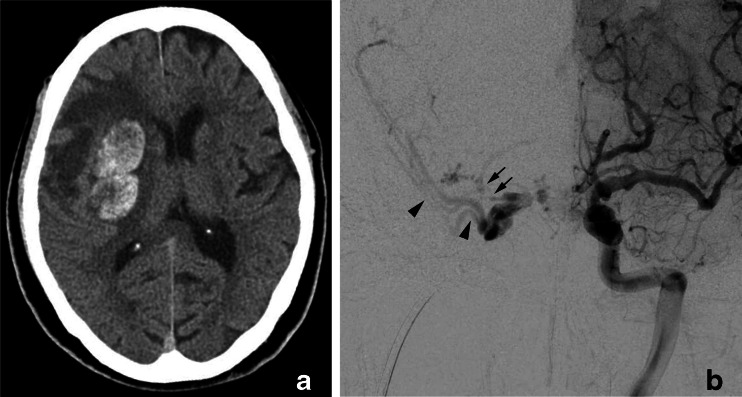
Cavernous sinus dural arteriovenous fistula with uncal venous drainage directly from the cavernous sinus. a CT shows right putaminal hemorrhage with perifocal edema. b Left internal carotid angiography shows the dural arteriovenous fistulas involving the right cavernous sinus. The AVFs drain into the superficial middle cerebral vein (*arrow heads*) and the uncal vein (*arrows*) directly from the right cavernous sinus
